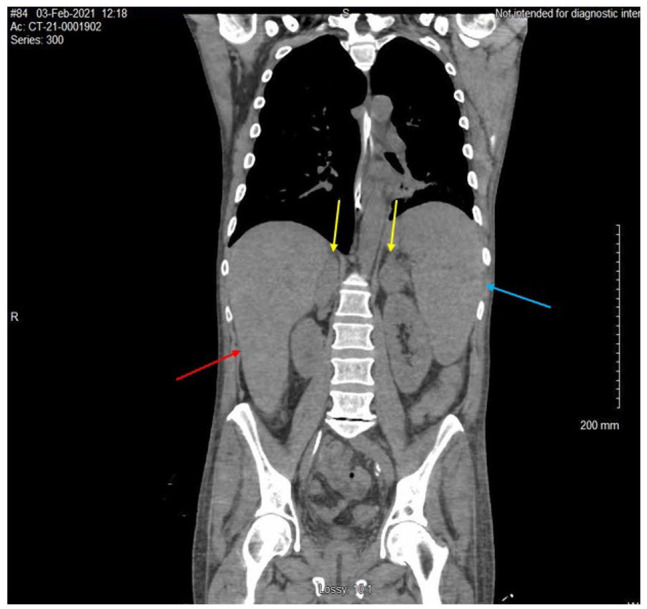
Computed tomography (CT) without contrast of the abdomen and pelvis revealed hepatomegaly with a craniocaudal length of 21 cm (red arrow), splenomegaly with a length of 18.2 cm (blue arrow), bilateral adrenal masses measuring 4 cm each (yellow arrow), and enlarged pericaval lymph nodes with a length of 2.3 cm × 1.7 cm × 4 cm.
